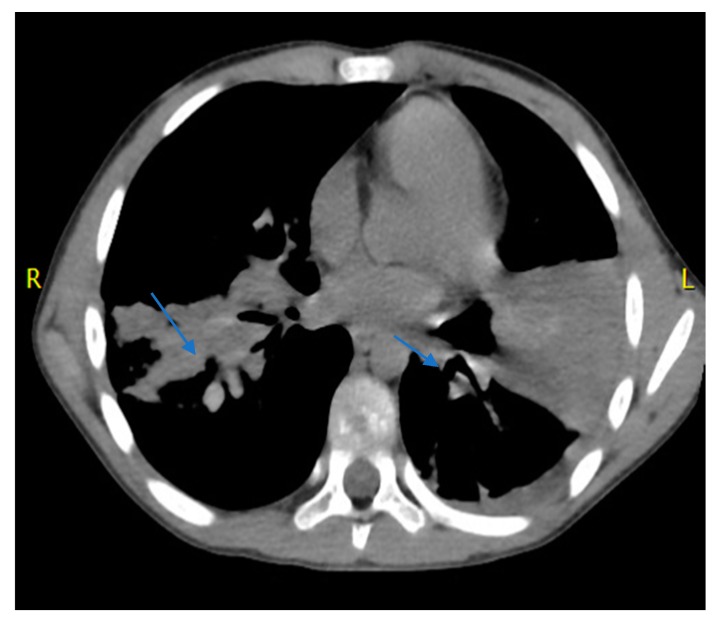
Computed tomography of bilateral bronchiectasis/bronchiolectasis with material in the context, suggesting high-attenuation mucus plugs.
